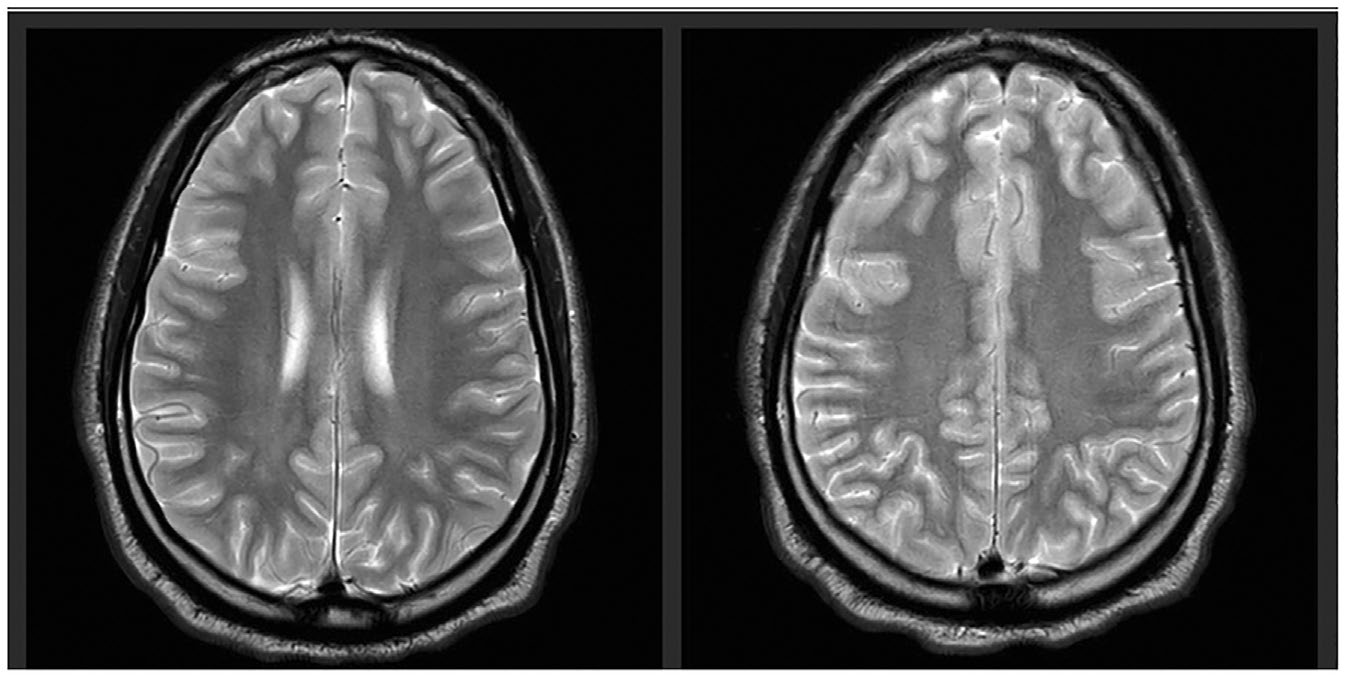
Axial T2‐weighted magnetic resonance images demonstrating cortical swelling with hyperintense T2 signal affecting frontal and parietal lobes, changes typical of those seen in the context of cerebral cortical encephalitis.
